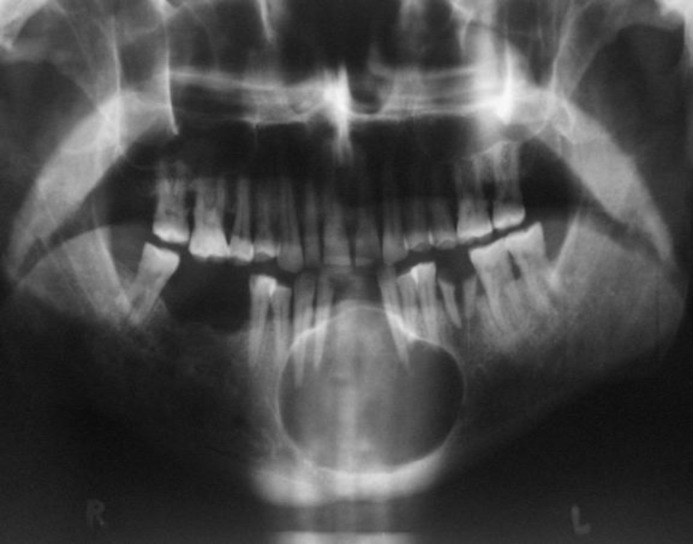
Panoramic radiography: a well-defined radiolucency with sclerotic borders between the left first molar and the right first premolar.
